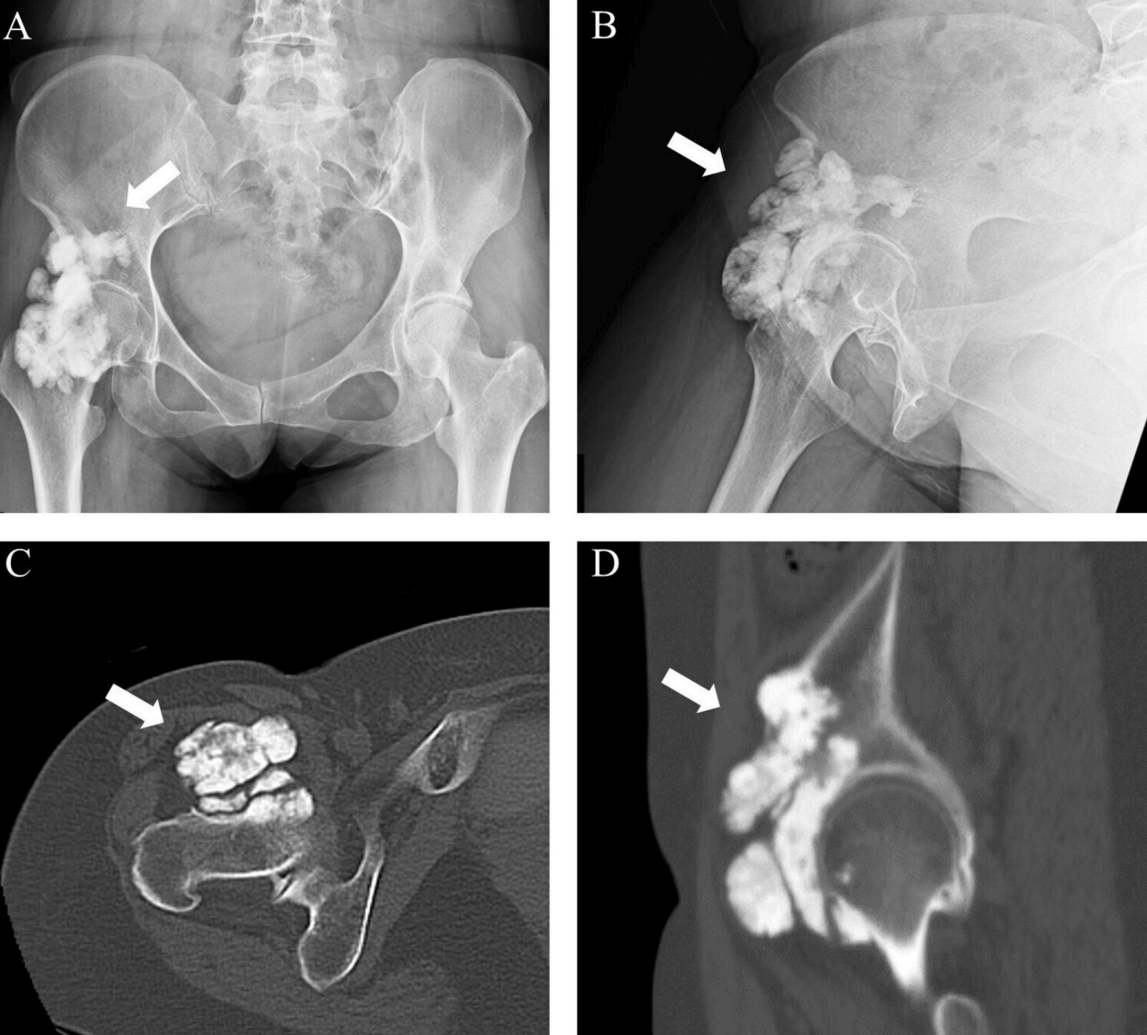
Plain radiographs of the right hip in anteroposterior position (A) and lateral position (B). Computed tomography (CT) of the right hip in the axial (C) and sagittal (D) views. The white arrow indicates the position of the mass. The mass was located anterior to the hip joint.
